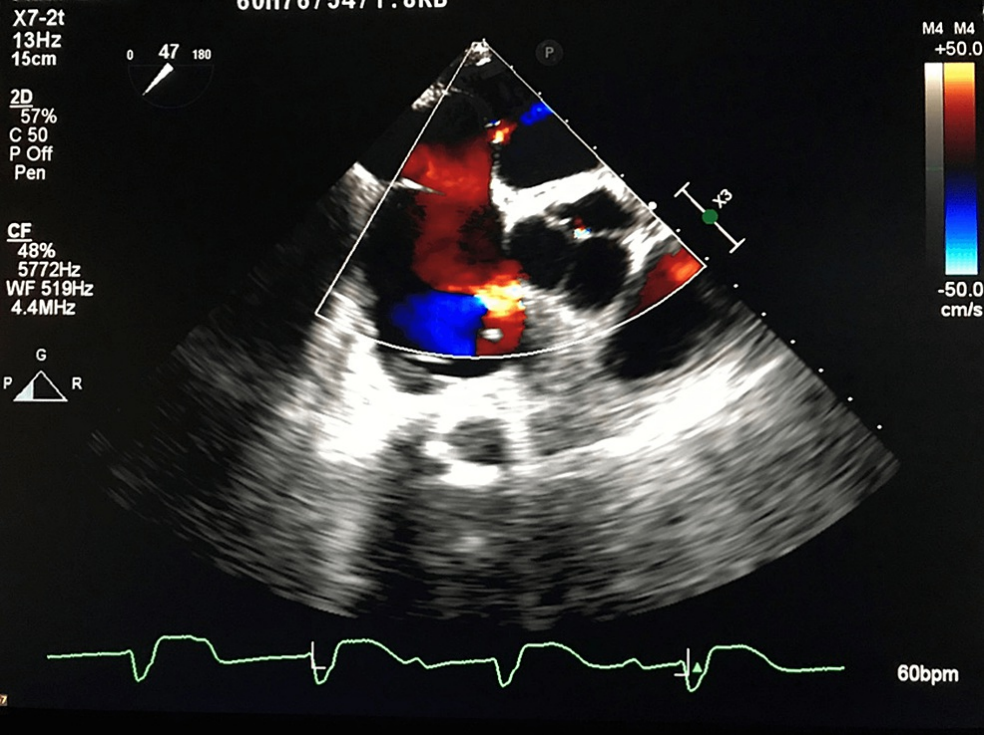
TEE visualization of ASD. TEE: transesophageal echocardiogram; ASD: atrial septal defect
